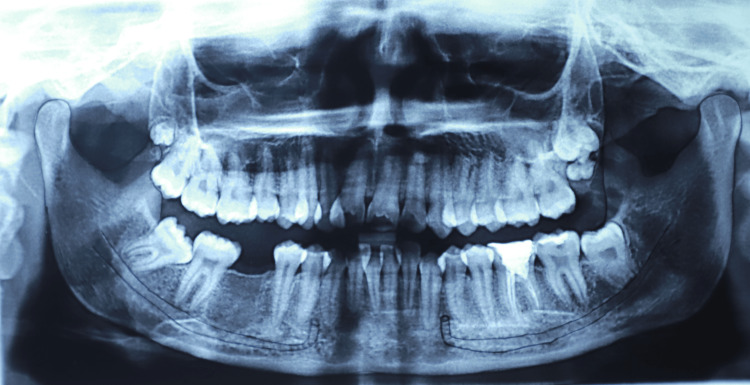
Tracing of panoramic radiograph showing the perpendicular pattern One of the digital panoramic images included in this study.
